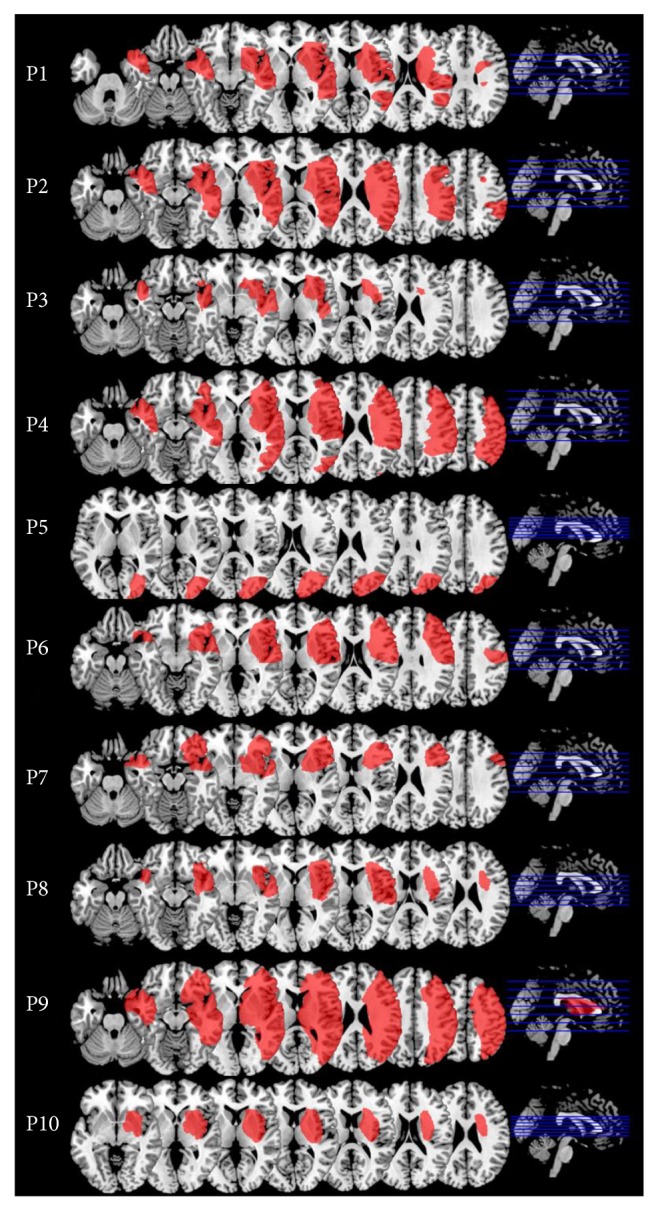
Lesions of individual patients displayed on axial slices of a normalized MRI template (positions of the slices in blue).
